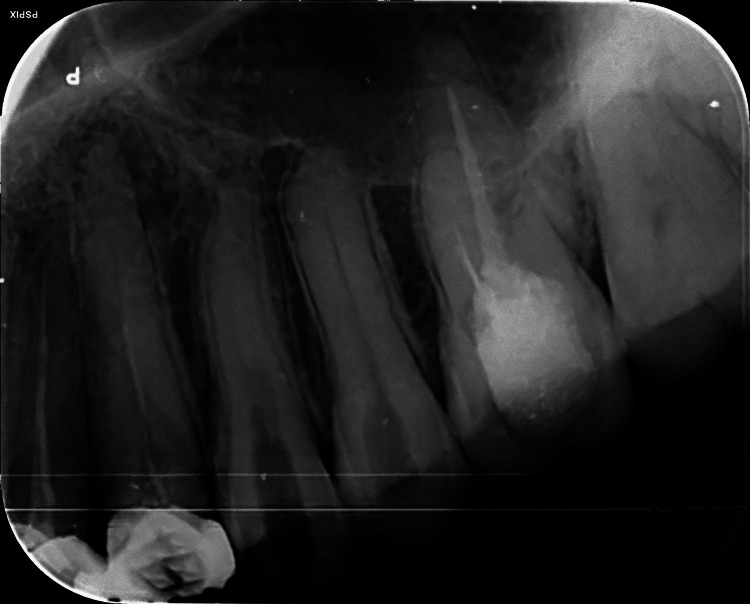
Postoperative radiograph after the removal of the separated fragment in 24 Confirmation of the retrieval of the separated fragment in 24 was done using a radiograph
